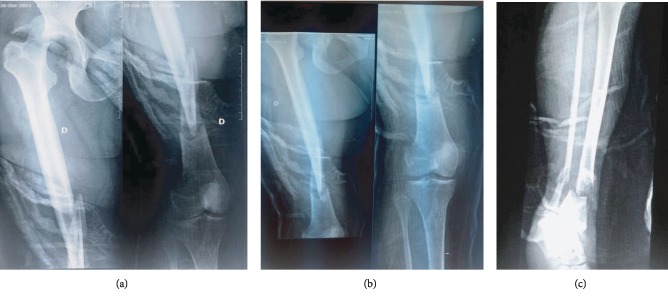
Initial radiographs of the fracture sites. (a, b) Radiographs showing dislocation of the right hip, displaced posterior wall of acetabular fracture, and concomitant femur shaft fracture. (c) Radiograph showing tibia fracture.
